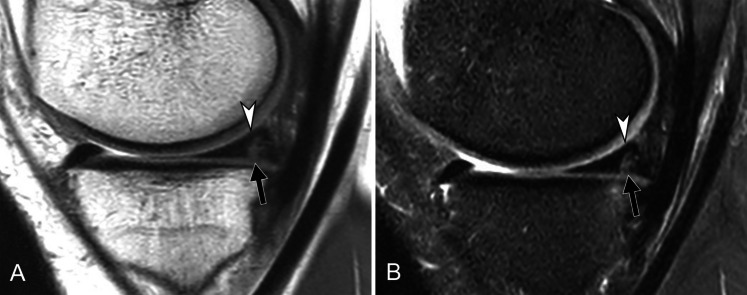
Sagittal proton-density-weighted MRI (A) and T2-weighted fat-suppressed MRI (B) of a 27-year-old man with ACL tear demonstrate partial-thickness longitudinal-vertical tear of the posterior horn of the medial meniscus (arrows) sparing the superior surface of the meniscus (arrowheads)
